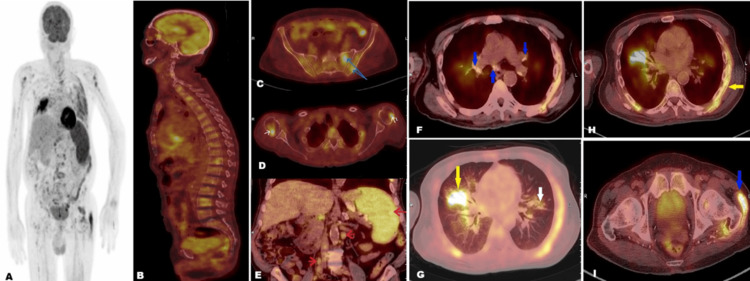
PET-CT findings Whole-body FDG PET images. Maximum intensity projection (A) and fused PET-CT images (B-I) showing patchy marrow activity in spines, pelvic bones (blue arrows in panel C) and humeri (white arrows in panel D), splenomegaly with enhanced FDG activity and active FDG-avid abdominal lymph nodes (red arrows in panel E), inactive calcified mediastinal nodes (blue arrows in panel F), right middle lung lobe consolidation (yellow arrow in panel G), peri-fissural nodular infiltrates (white arrow in panel G), myositis ossificans at left chest wall (yellow arrow in panel H) and at lateral aspect of left femoral trochanter (blue arrow in panel I) PET-CT: positron emission tomography/computed tomography; FDG: fluorodeoxyglucose
